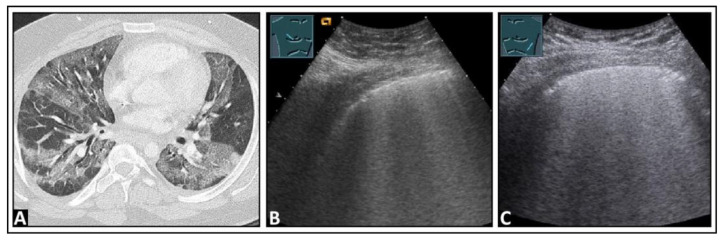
A patient with confirmed COVID-19 infection with progressive respiratory failure. (A) The computed tomography scan displays extensive bilateral ground-glass opacities, in some areas also emphasizing the interlobular septa (crazy paving), consistent with COVID-19 pneumonia. (B) On lung ultrasound, both on the right side and (C) on the left side, there are multiple confluent B-lines, indicating a “white lung” pattern consistent with a diffuse interstitial pattern.
